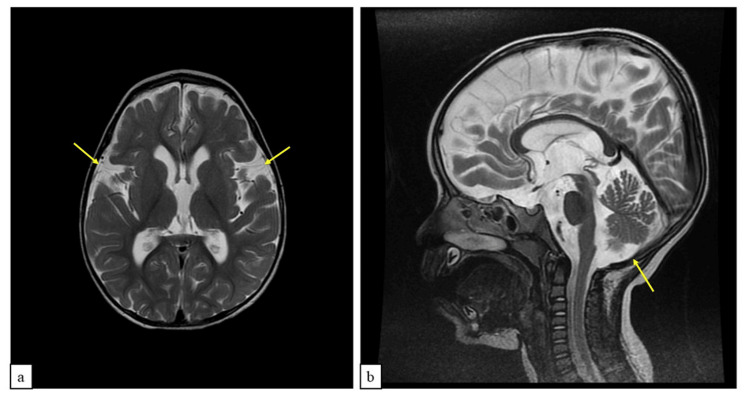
T2 MRI images of the child showing prominent cerebellar folia and brain volume reduction a. Axial view. b. Sagittal view.
